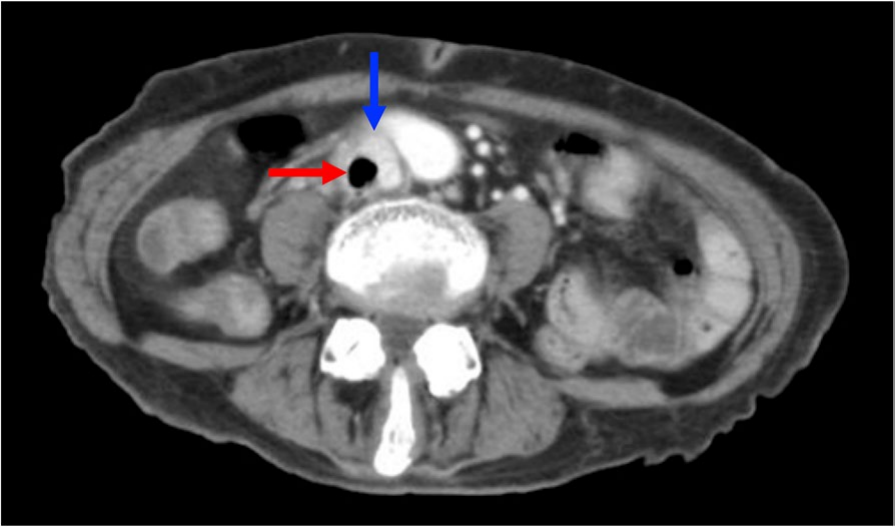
Axial contrast-enhanced computed tomography (CECT) showing round gas in a thrombus (red arrow) in the inferior vena cava (blue arrow)
